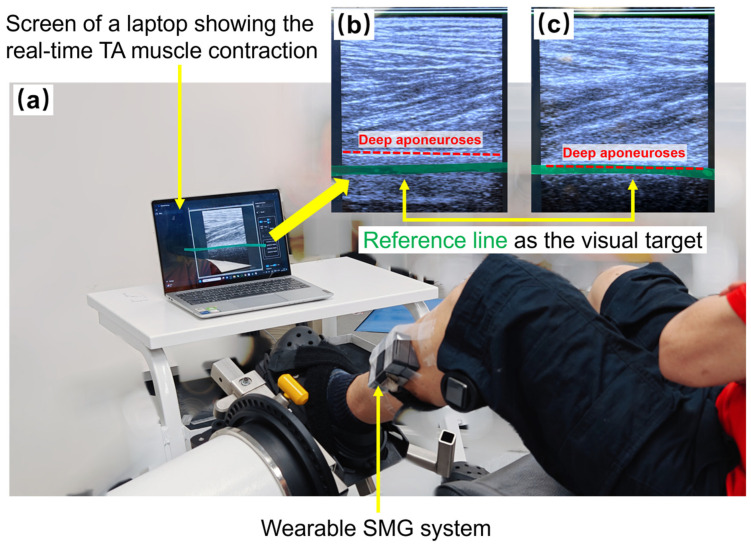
Experimental setup of ultrasound-imaging-based visual feedback (UVF) muscle training. (a) Placement of wearable ultrasound probe and display for UVF; (b) an example of the captured ultrasound image showing the TA muscle during resting phase; (c) an example of the captured ultrasound image showing the TA muscle during isometric contraction. Note: SMG—sonomyography; TA—tibialis anterior.
